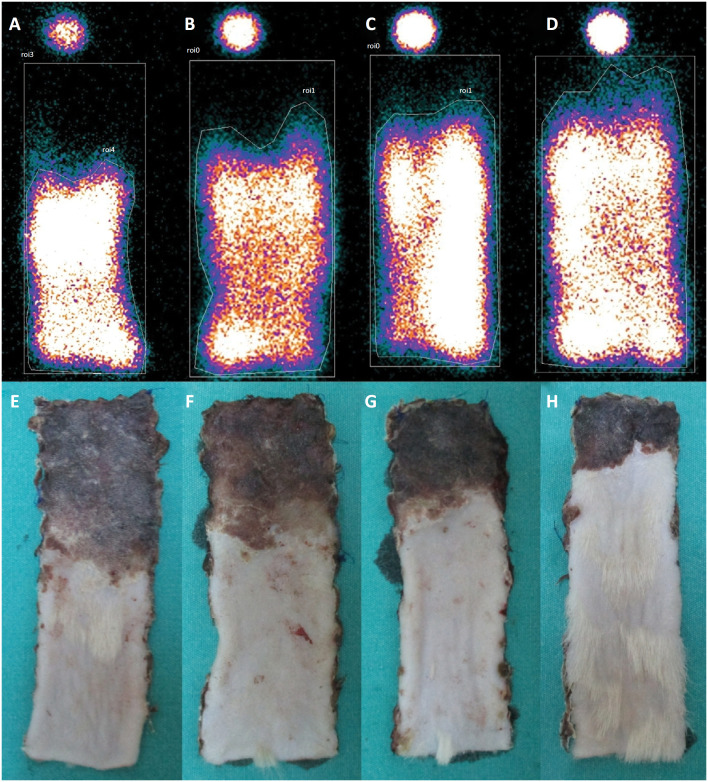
The scintigraphic perfusion images of the flaps of a)
